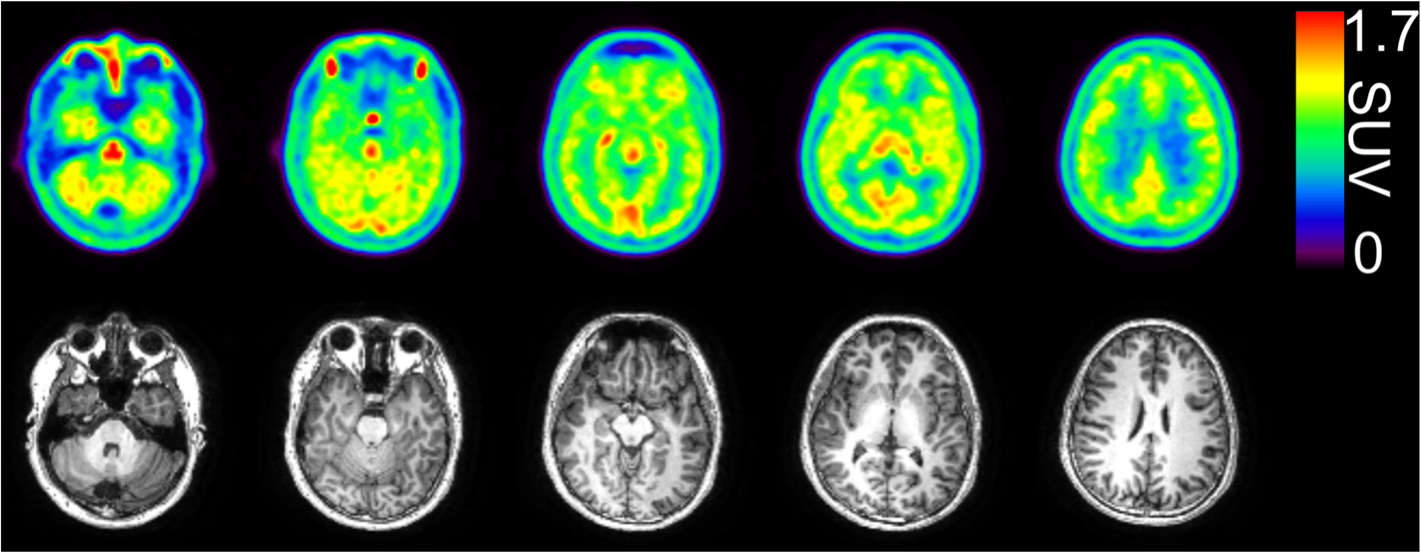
Representative magnetic resonance and static images of [^11^C]CB184 PET obtained from a 28-year-old male subject. (*Upper*) [^11^C]CB184 PET images (SUV summed 40–60 min). (*Lower*) Magnetic resonance images. PET images were smoothed with a Gaussian filter of 4 mm in FWHM
